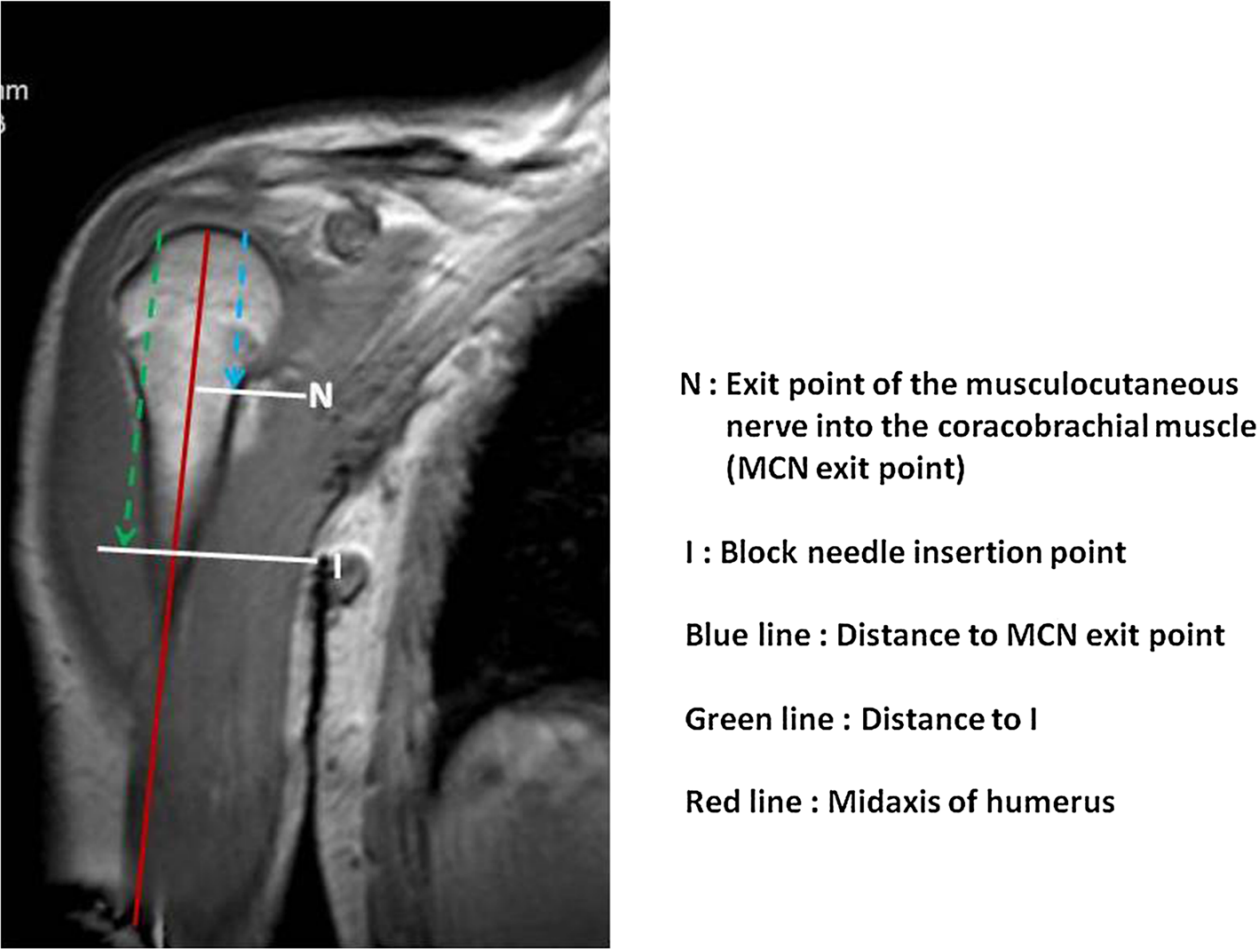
MRI of right shoulder, coronal view, in one of the patients. T1-weighted image. With a reference point at the top of the humeral head, the distances (*blue*
